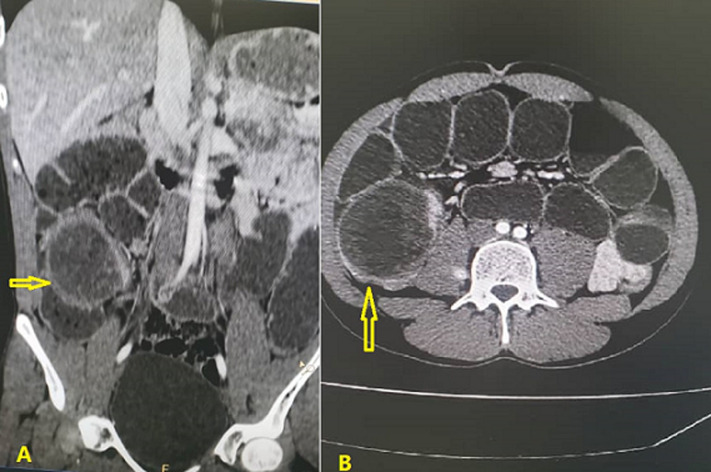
A) coronal CT scan showing the cecal duplication cyst (arrow); B) axial CT shows a non-communicating cecal duplication cyst measuring 8.8x7.5x6 cm (arrow) and dilated small bowel
